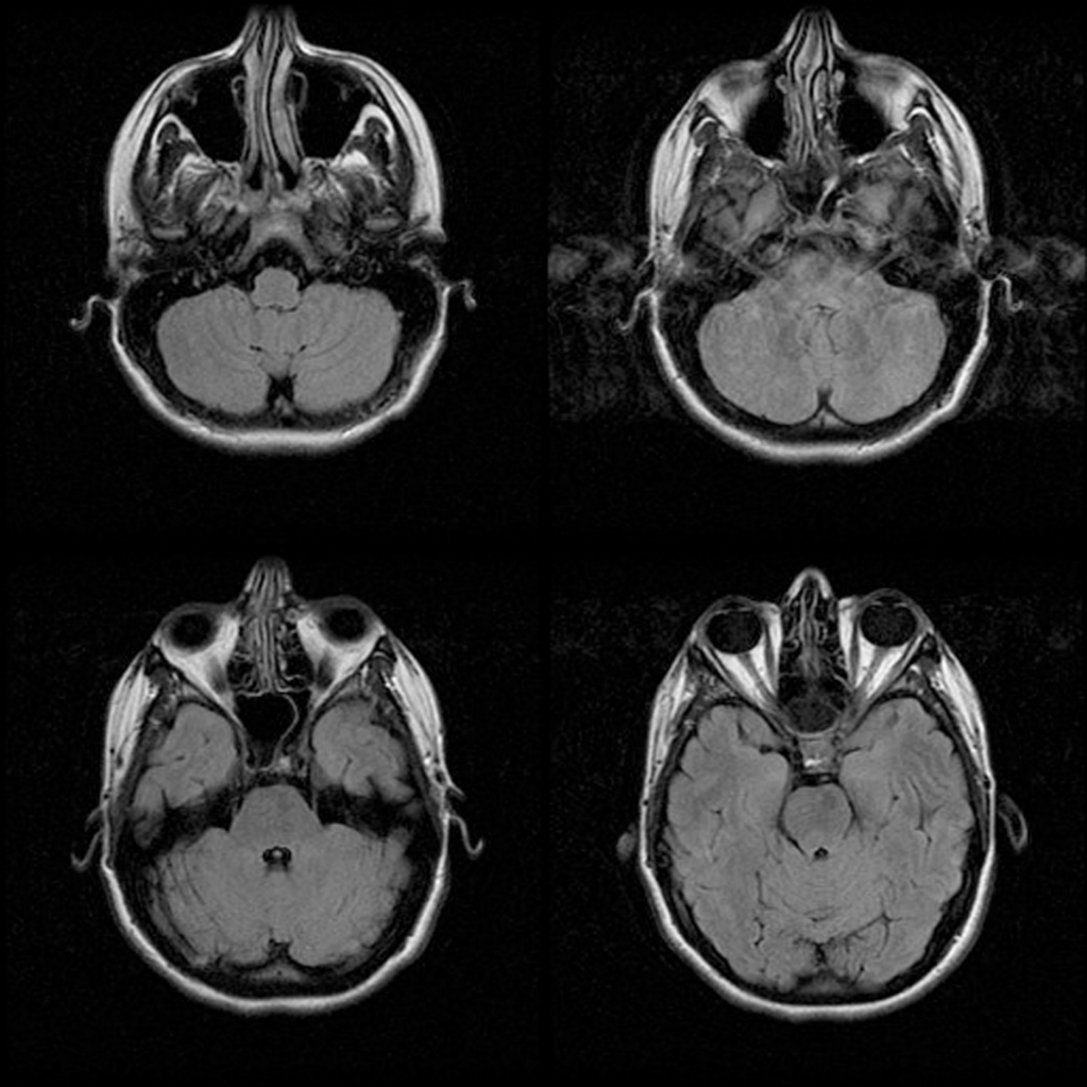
MRI Images Axial images, T2 weighted with Flair. "There has been near resolution of the mucosal disease within the paranasal sinuses with only minimal mucosal thickening remaining within the sphenoid sinuses and ethmoid air cells bilaterally. Significant improvement in the intracranial extent of disease. There has been significant improvement in the degree of inflammatory leptomeningeal enhancement with mild residual enhancement remaining within the suprasellar cistern.” MRI: magnetic resonance imaging; Flair: fluid attenuated inversion recovery
